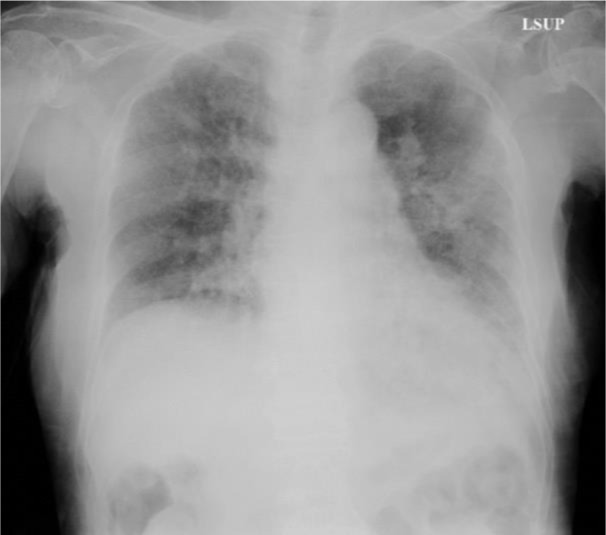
Chest radiography on admission showed bilateral reticular shadows but no finding of cavity lesions.
